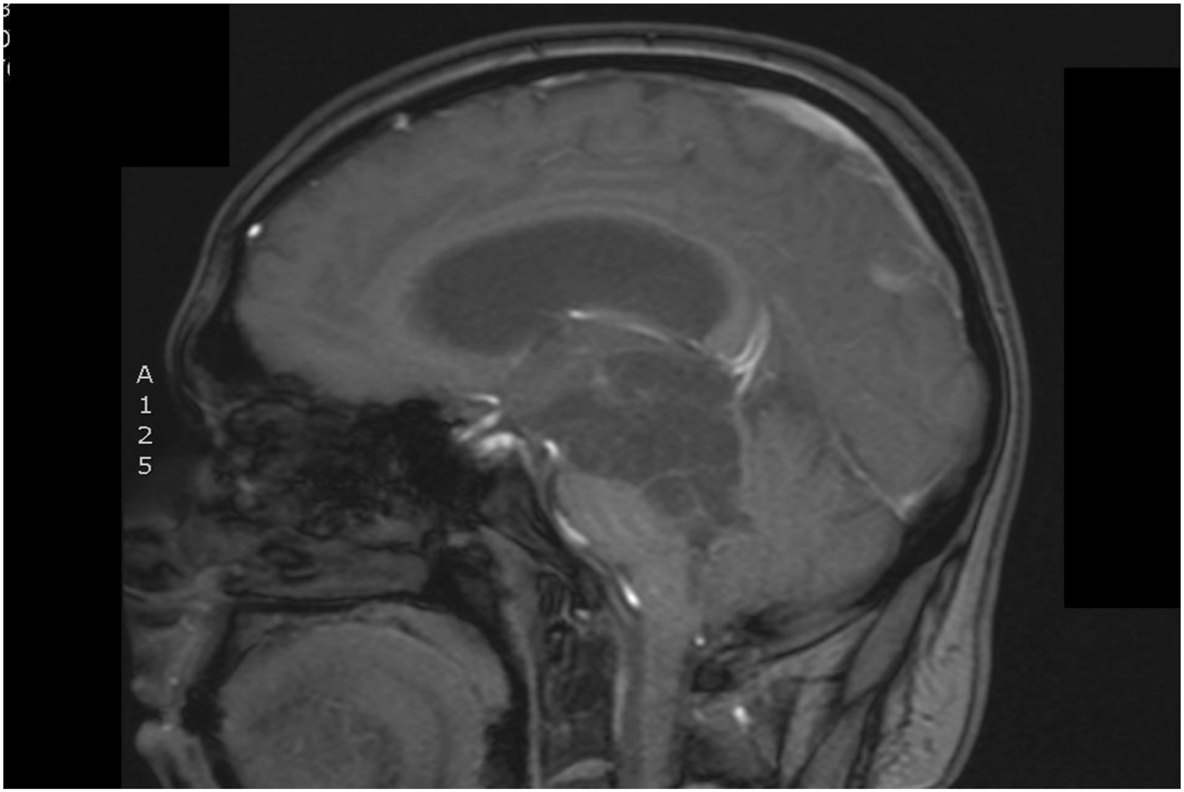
Magnetic resonance image of the brain showing the complex cystic lesion extending from the left hemipons to the thalamus.
